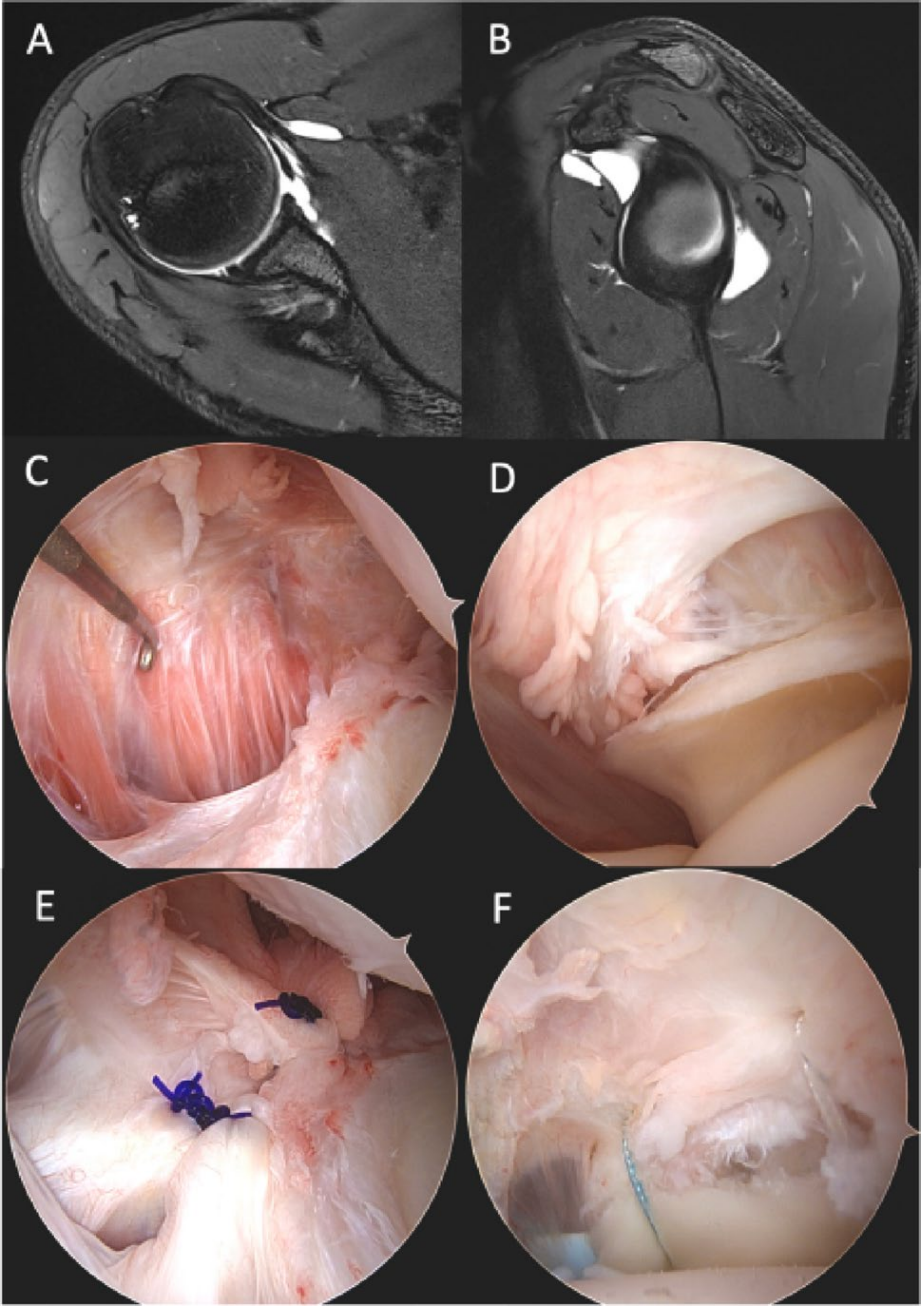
A 14-year-old male patient presented with anterior instability of the right shoulder. Pathologies identified in axial (A) and sagittal (B) MRI scans included an anteroinferior intracapsular rupture (C), and an articular-sided mid-substance partial tear of the supraspinatus tendon (D), all confirmed during the diagnostic round. Surgical management involved side-to-side closure of the capsular rupture (E), and reconstruction of the partial supraspinatus tendon tear (F)
